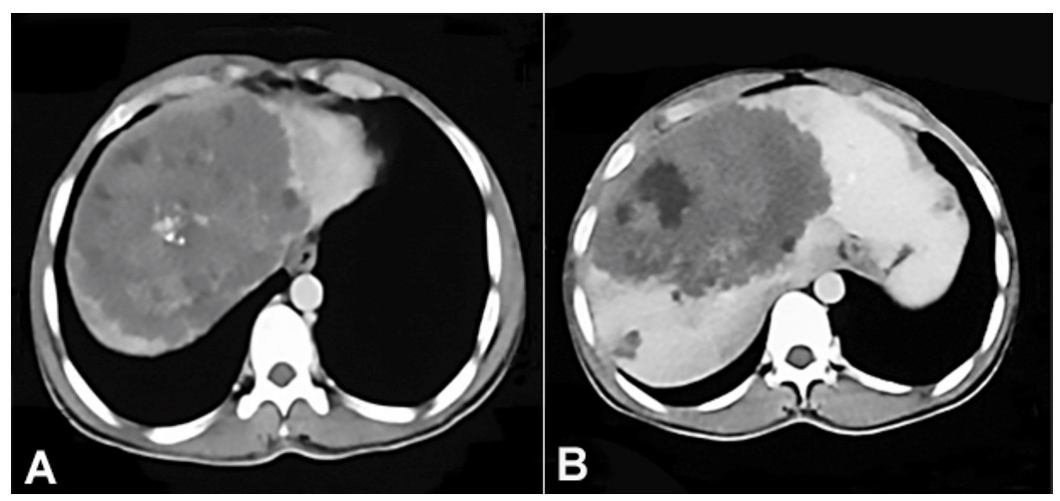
Contrast-enhanced computed tomography (CECT) revealing a large solid-cystic intrahepatic lesion with A - a few specks of calcification and B - central hypodense areas of necrosis.
